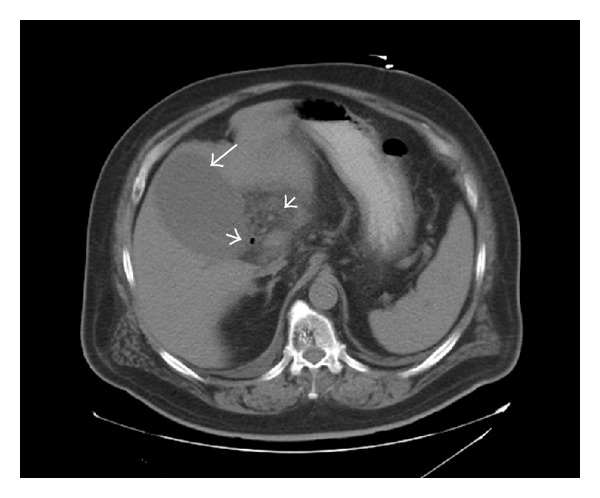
Computed tomography of the abdomen with oral contrast showing the gallbladder markedly distended (long arrow). Air bubbles project over the region of gallbladder neck are seen, suggestive of infection with gas-producing organism (short arrows).
